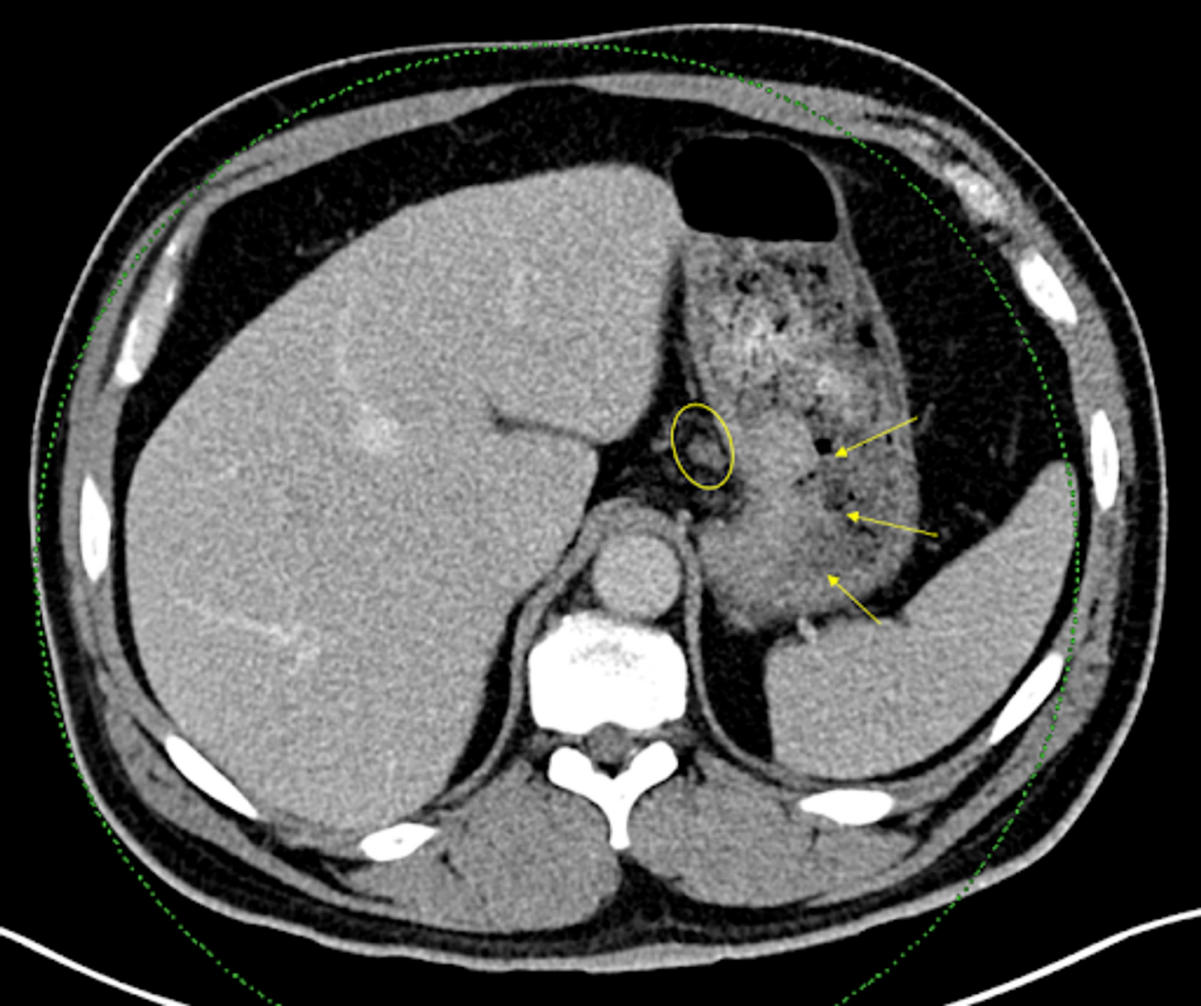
CT scan of the abdomen. The yellow arrows depict a large polypoid soft tissue mass arising from the lesser curvature of the stomach extending posteriorly towards the gastric cardia measuring at least 3.4 cm x 6.0 cm, without extension into distal esophagus or through the wall of the stomach. The yellow circle depicts one prominent peri-gastric lymph node measuring 11 mm, indeterminate for metastatic disease.
